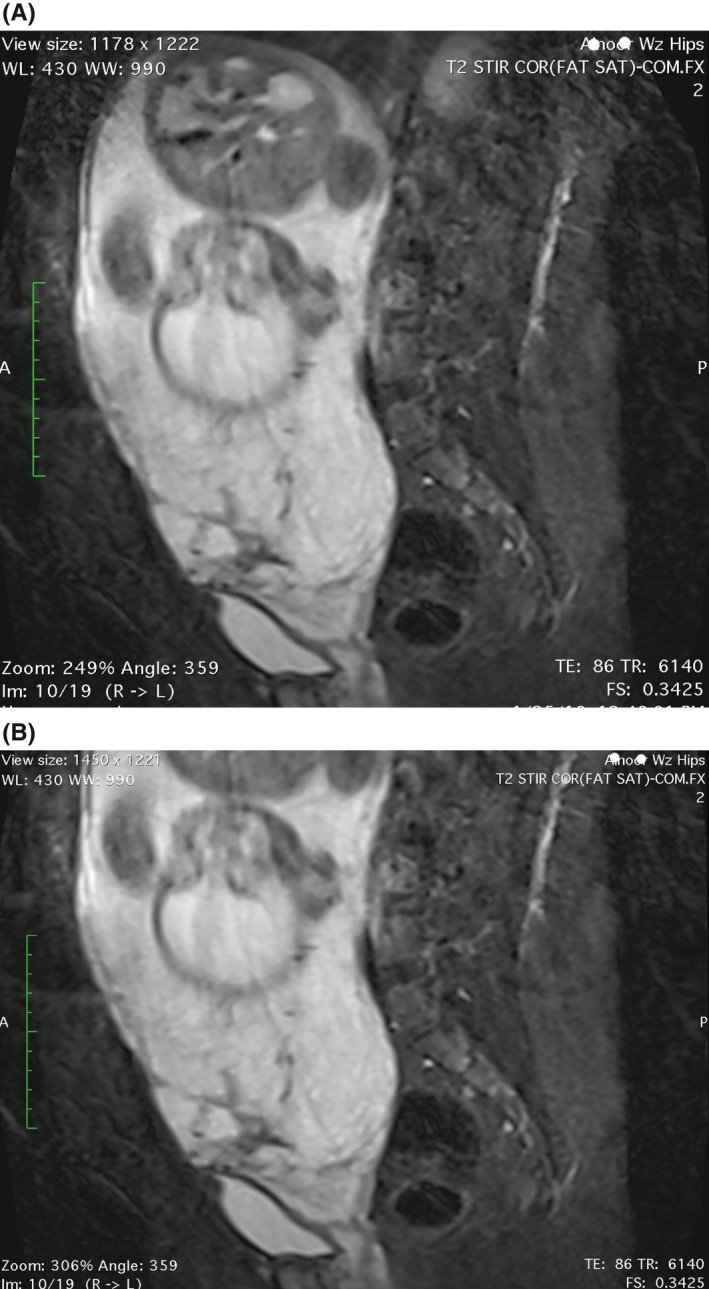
Magnetic resonance imaging (MRI) Pelvis without contrast: (A) sagittal T2‐weighted MR image shows bulging of the uterus and tenting of the bladder. (B) Placenta lies anteriorly along with lower uterine segment completely covering the internal os
